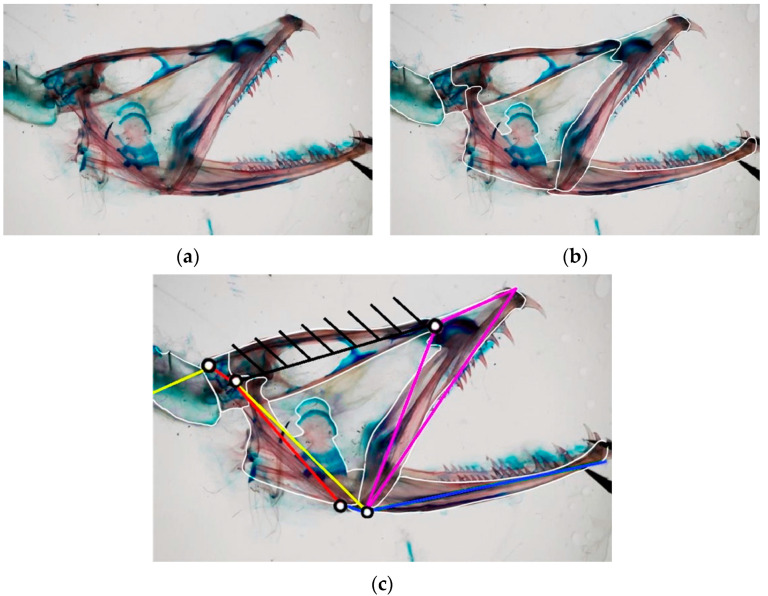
(a) Radiography of Eustomias obscurus [29]; (b) radiography of Eustomias obscurus with bodies highlighted; (c) radiography of Eustomias obscurus with bodies highlighted and corresponding linkage mechanism.
